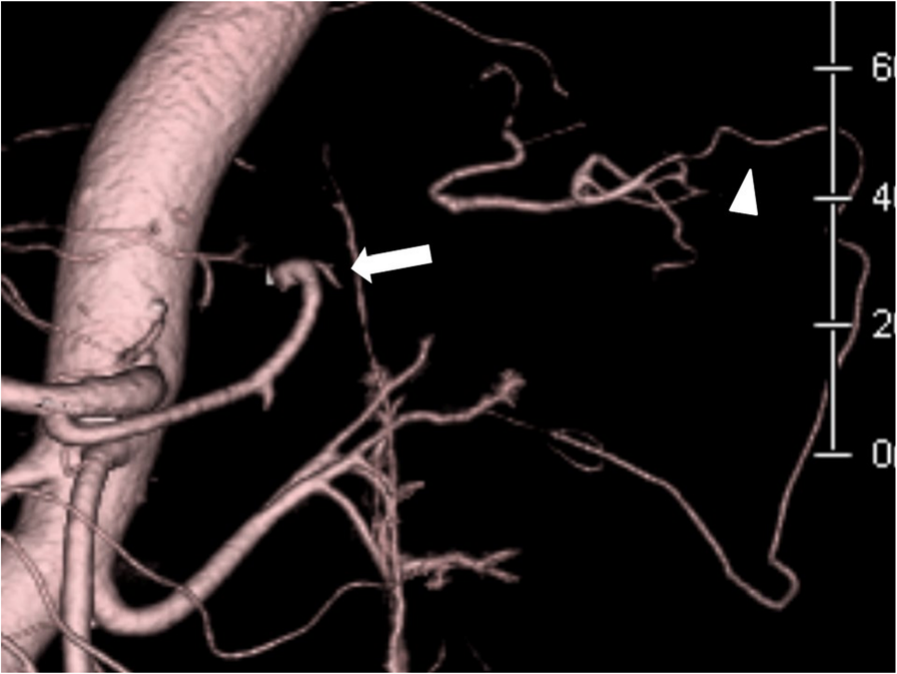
Postoperative enhanced computed tomography. Postoperative enhanced computed tomography reveals the arteries that could supply blood to the spleen.*White triangle: omental branches of the left gastroepiploic artery via the omental artery; white arrow: the great pancreatic artery via the pancreas
